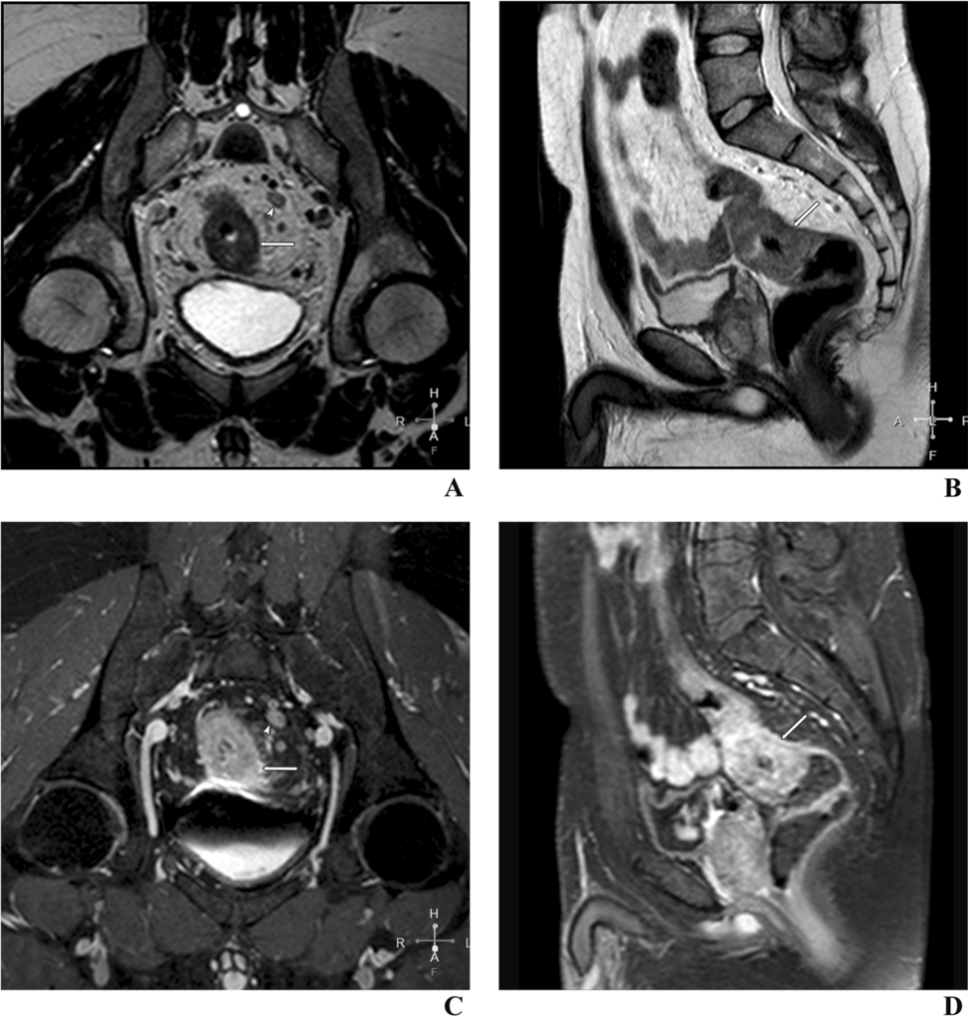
Rectal cancer with extramural extension in perirectal fat (arrow) and metastasis in a lymph node (arrowhead). Examination with a 1.5-T pelvic phased array coil. Oblique T2- (A) and T1-weighted with fat suppression post-gadolinium-enhanced images (C). Sagittal T2- (B) and T1-weighted with fat suppression post-gadolinium-enhanced images (D).
